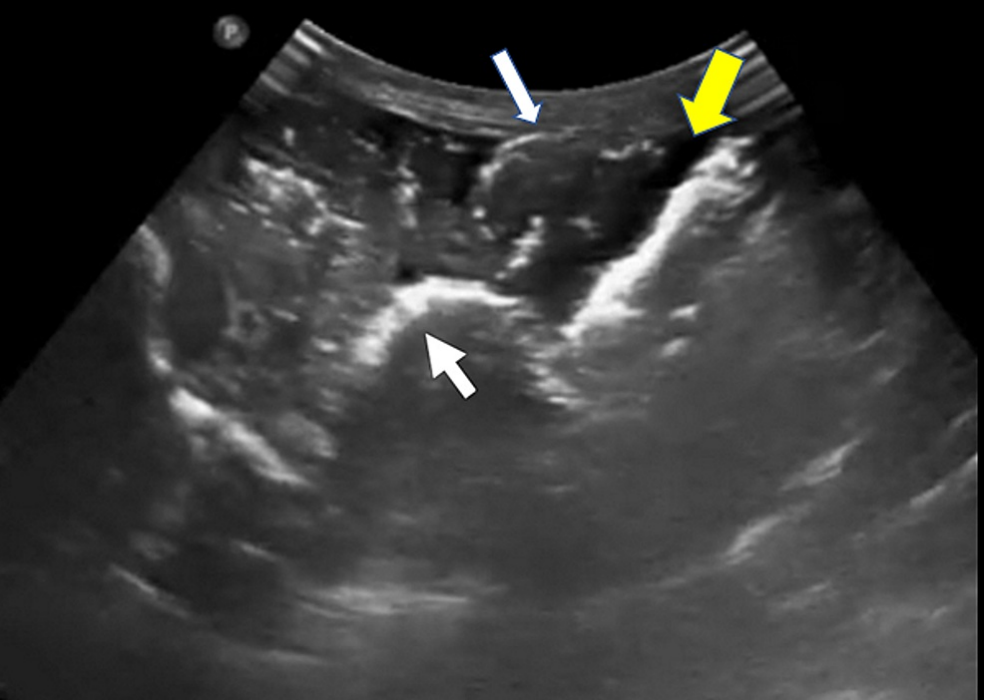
Bedside ultrasound image obtained from the lower abdomen (transverse view) using curvilinear probe, demonstrating edematous bowel wall with intra-mural air which appeared as hyper-echoic lining along the bowel wall (white arrows) suggestive of pneumatosis intestinalis. There was also free fluid demonstrated in the ultrasound image as shown by the yellow arrow. White arrows: intra-mural air which appeared as hyper-echoic lining along the bowel wall suggestive of pneumatosis intestinalis. Yellow arrow: Free fluid
